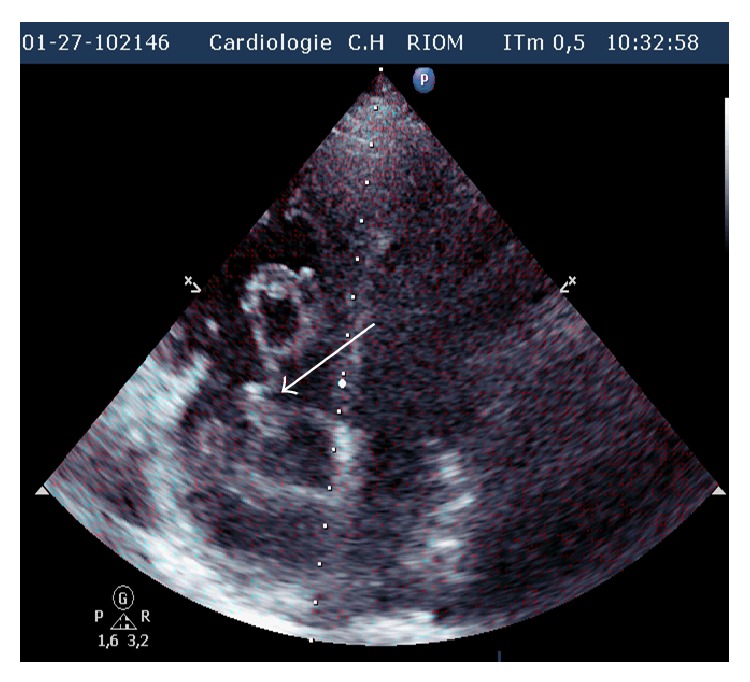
Apical four chamber view showing a serpiginous thrombus (white arrow) in the right heart chambers.
